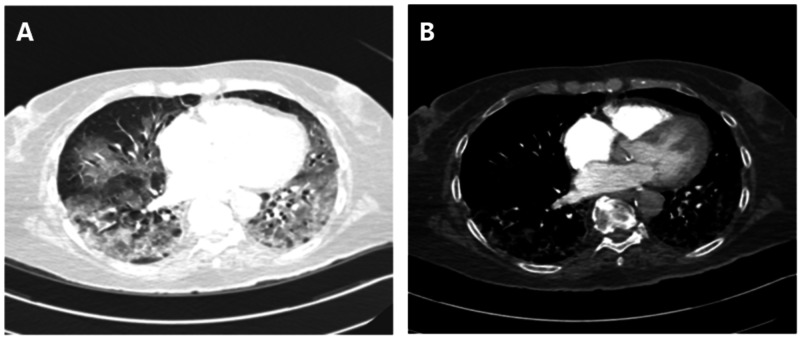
CTPA. A: Lung window demonstrates an appearance in the chest in keeping with classic COVID-19. B: Shows no central PE. CTPA, CT pulmonary angiogram; PE, pulmonary embolism
